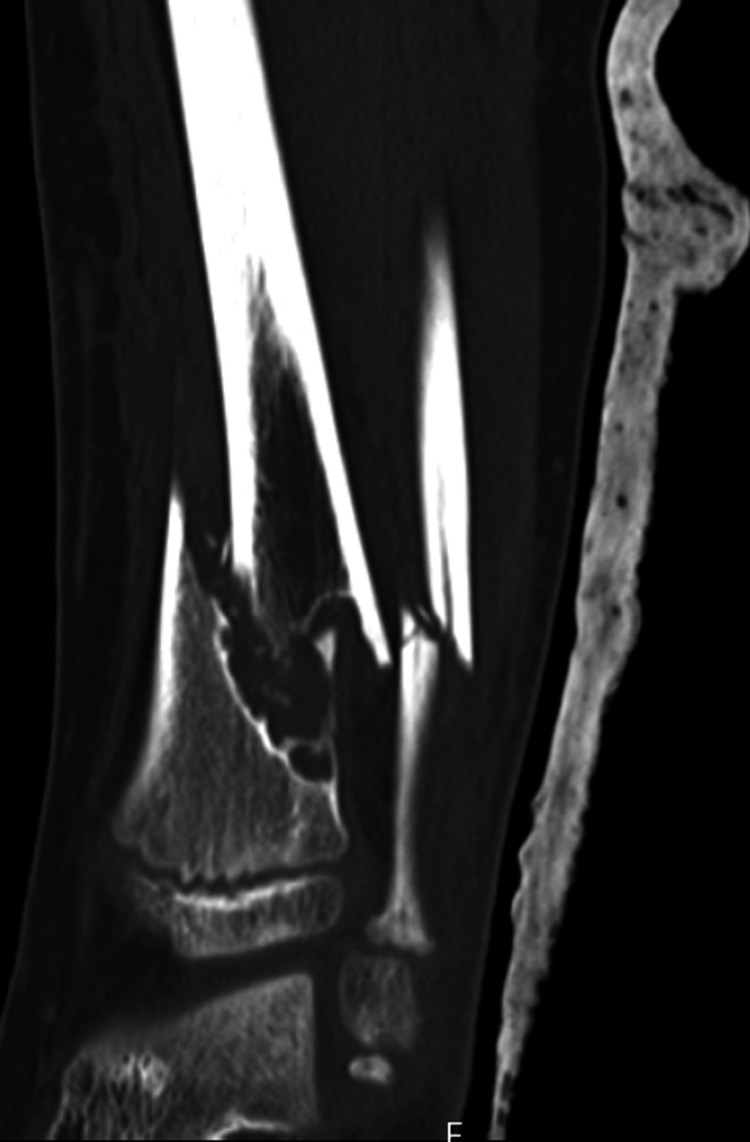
Coronal CT view of the left tibia and fibula
